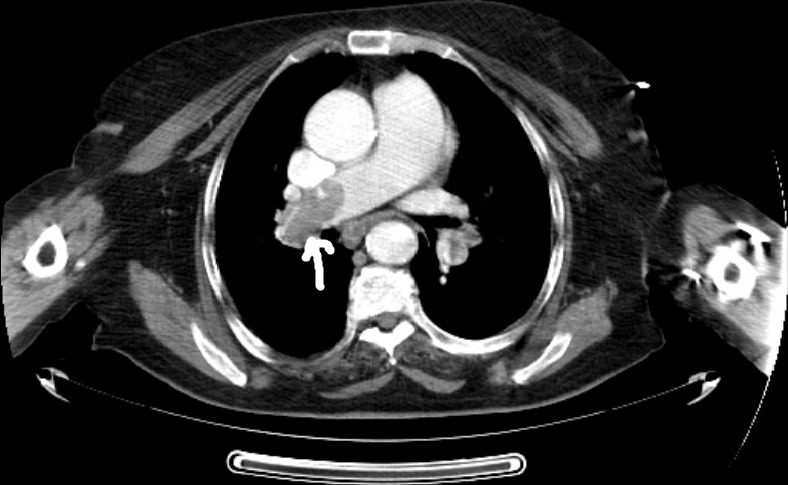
Pulmonary CT angiography with contrast demonstrates hypodense filling defect within the rigt main pulmonary artery consistent with pulmonary embolism
